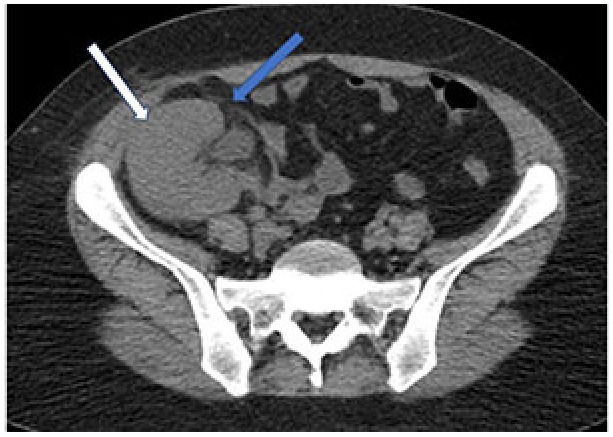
A 39-year-old female COVID-19 patient presenting with cough, fever for five days, and increased creatinine levels. CT scan shows the increased size in the transplanted kidney (white arrow) and increased density consistent with inflammation in the perirenal fatty tissue (blue arrow).
